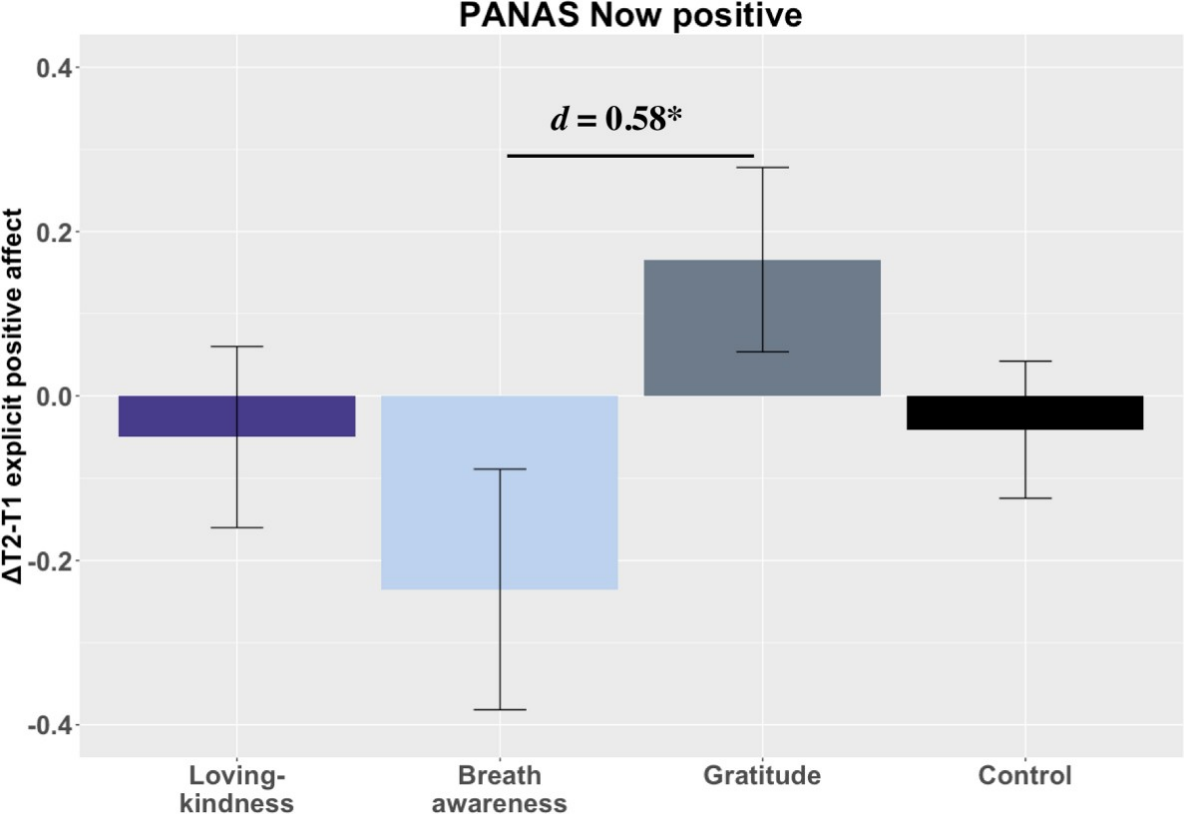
Training changes in positive affect. Notes: * = *p* < .001, * = p* < .01,  = p <* .05, *t = p* < .10. Loving-kindness = LK; Breath awareness = BA; Gratitude = GT; Control = CT.
